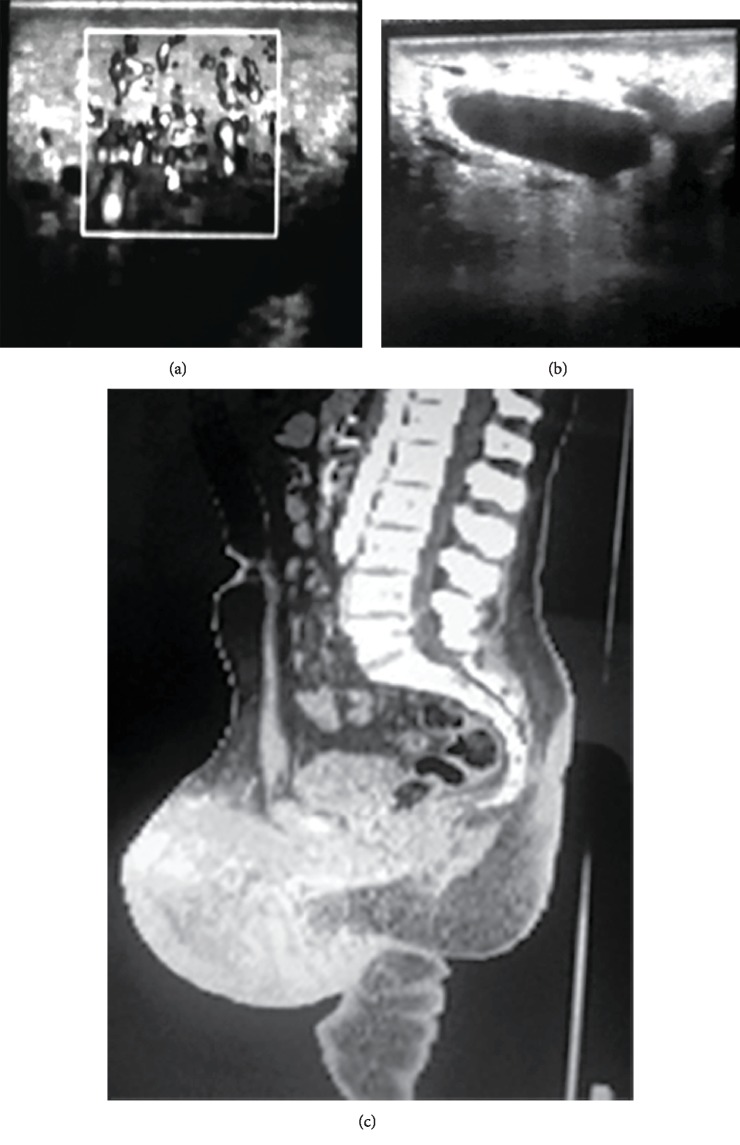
(a, b) Doppler ultrasound showed dilated vessels with both venous and arterial components; (c) computed tomography arteriography.
